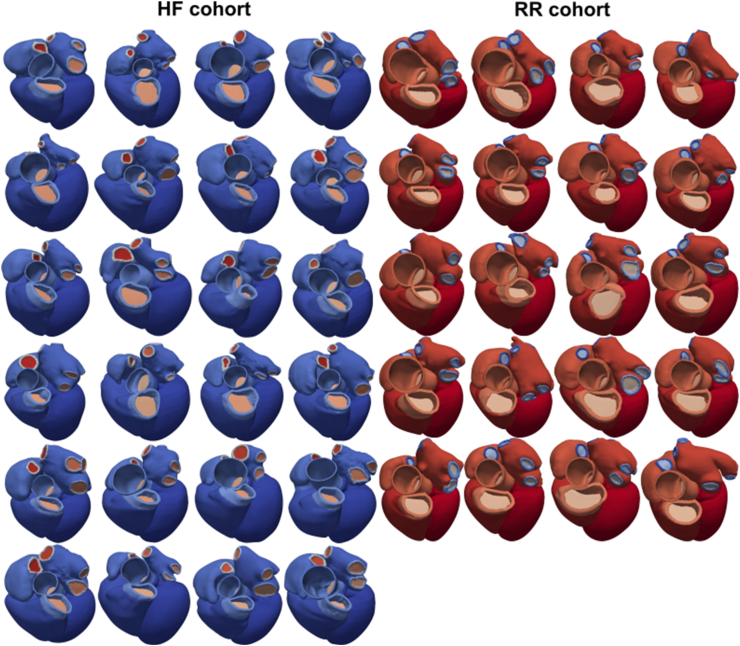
Four-chamber heart meshes from 24 HF patients (blue, left) and 20 RR (red, right) CT datasets. Only the ventricles were used in this study. (For interpretation of the references to colour in this figure legend, the reader is referred to the Web version of this article.)
